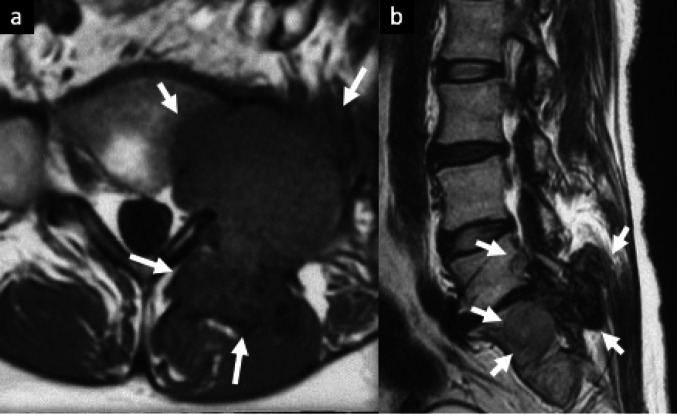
Malignant tenosynovial giant cell tumor arising in the facet joint. Axial T1-weighted MR image (a) demonstrates a low signal intensity mass with bony destruction involving the left L5/S1 facet joint (arrows). On T2-weighted sagittal MR image (b), low signal intensity in the posterior elements is more prominent (arrows)
